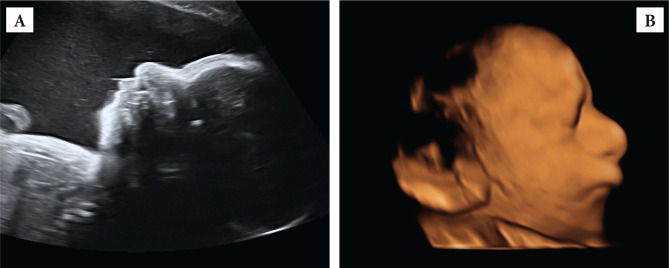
A – Ultrasound scan showing the abnormal fetal profile and polyhydramnios; B – 3D volumetric reconstruction of the fetal face showing atypical facial features and low set years.
